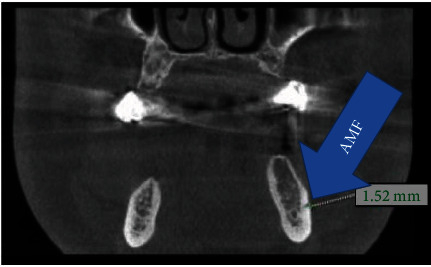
Left AMF at coronal CBCT.
